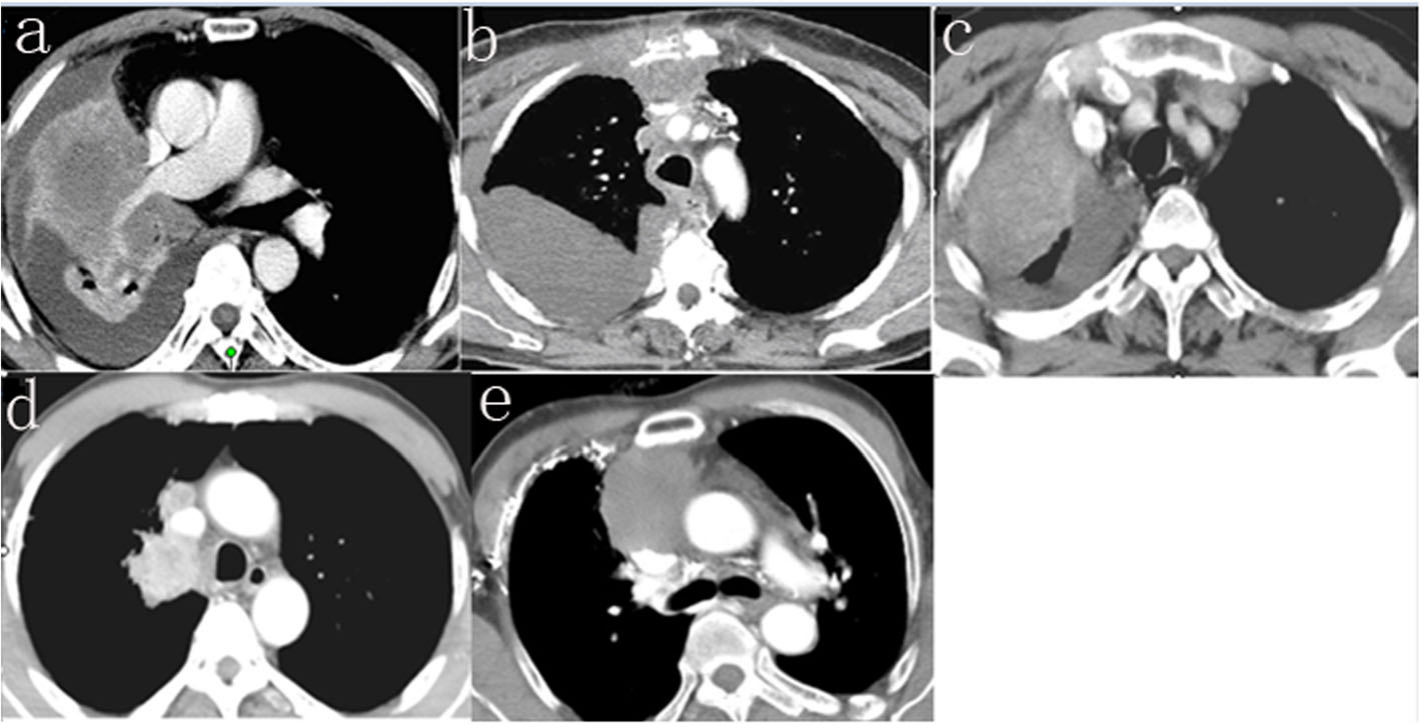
Tumors invading SVC on chest CT. a Right hilus pulmonis Sq.in patient 2. b Dysgerminoma in patient 3. c Right hilus pulmonis Sq.in patient 4. d Right upper lobe Sq.in patient 5. e Type AB thymoma in patient 6. *SVC* superior vena cava, *Sq.* squamous cell carcinoma, *CT* computed tomography
